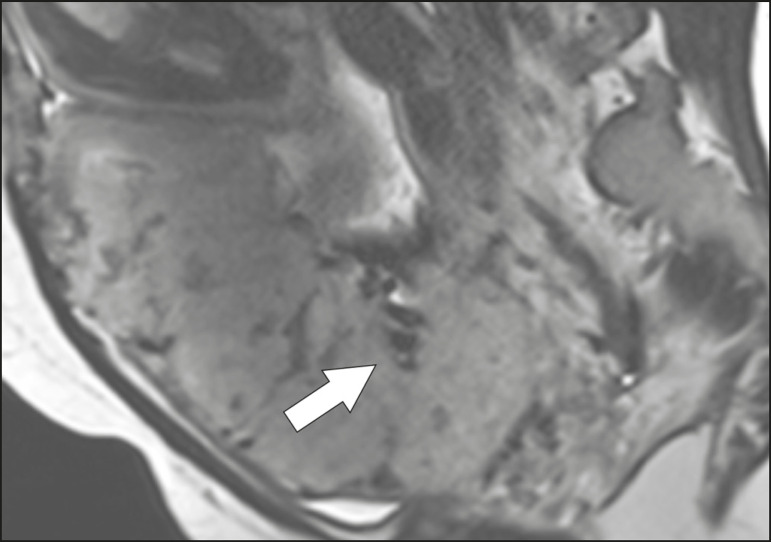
Sagittal T2-weighted MRI sequence showing complete placenta previa accompanied by disorganized placental vascularity and vessels with calibers going up to 9 mm (arrow).
